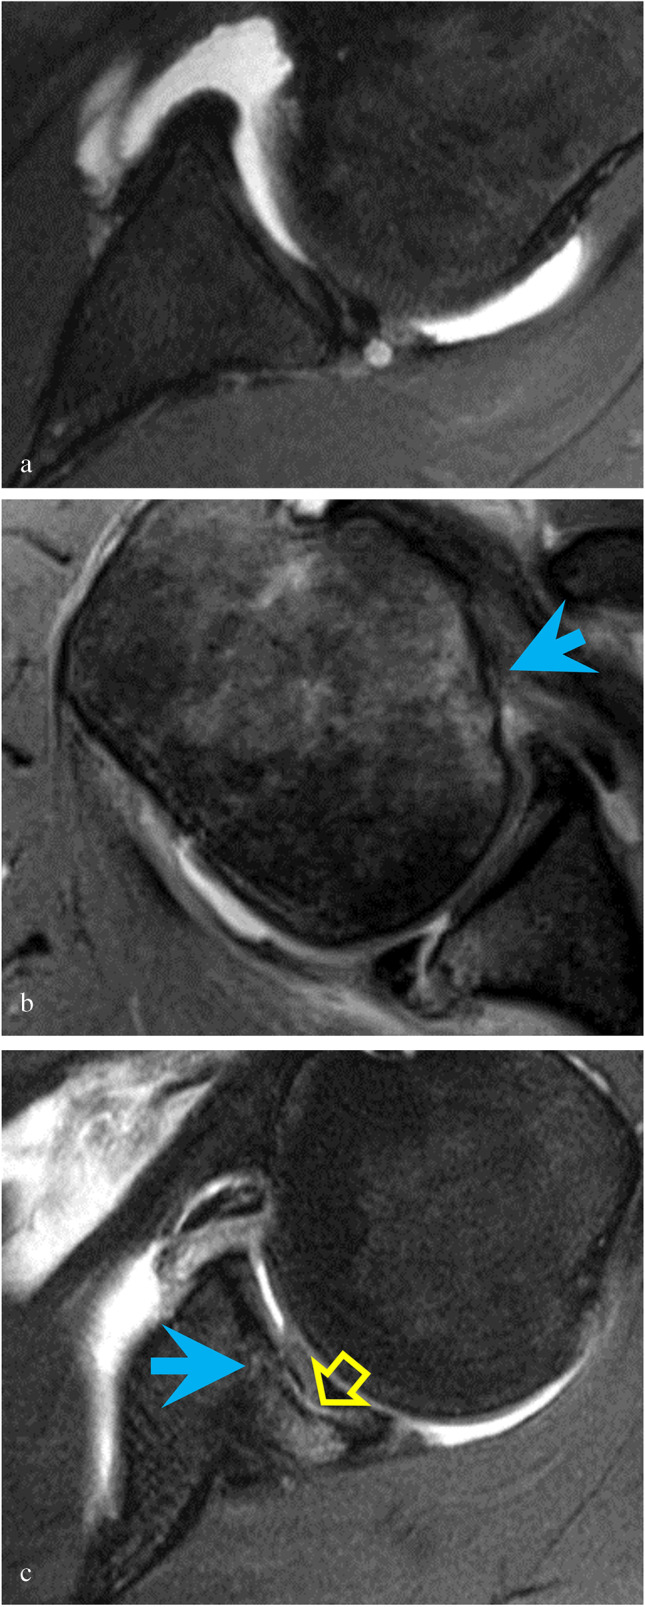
a Axial T2-weighted image with fat suppression shows a paralabral cyst in a 32-year-old male with an isolated posterior labral tear. b Axial T2-weighted image with fat suppression shows a humeral fracture (reverse Hill-Sachs) with cortical irregularity and flattening of the humeral head (blue arrow) in a 47-year-old male who suffered a posterior shoulder dislocation during a seizure and with 2 weeks of recurrent subluxations. c Axial T2-weighted image with fat suppression shows a glenoid fracture (reverse bony Bankart) with focal fracture and depression of the glenoid (blue arrow) and cartilage delamination (yellow open arrow) in a 31-year-old male former softball player who at the time of imaging unloaded heavy parcels at work. The osteochondral injury was noted to be unstable at surgery
